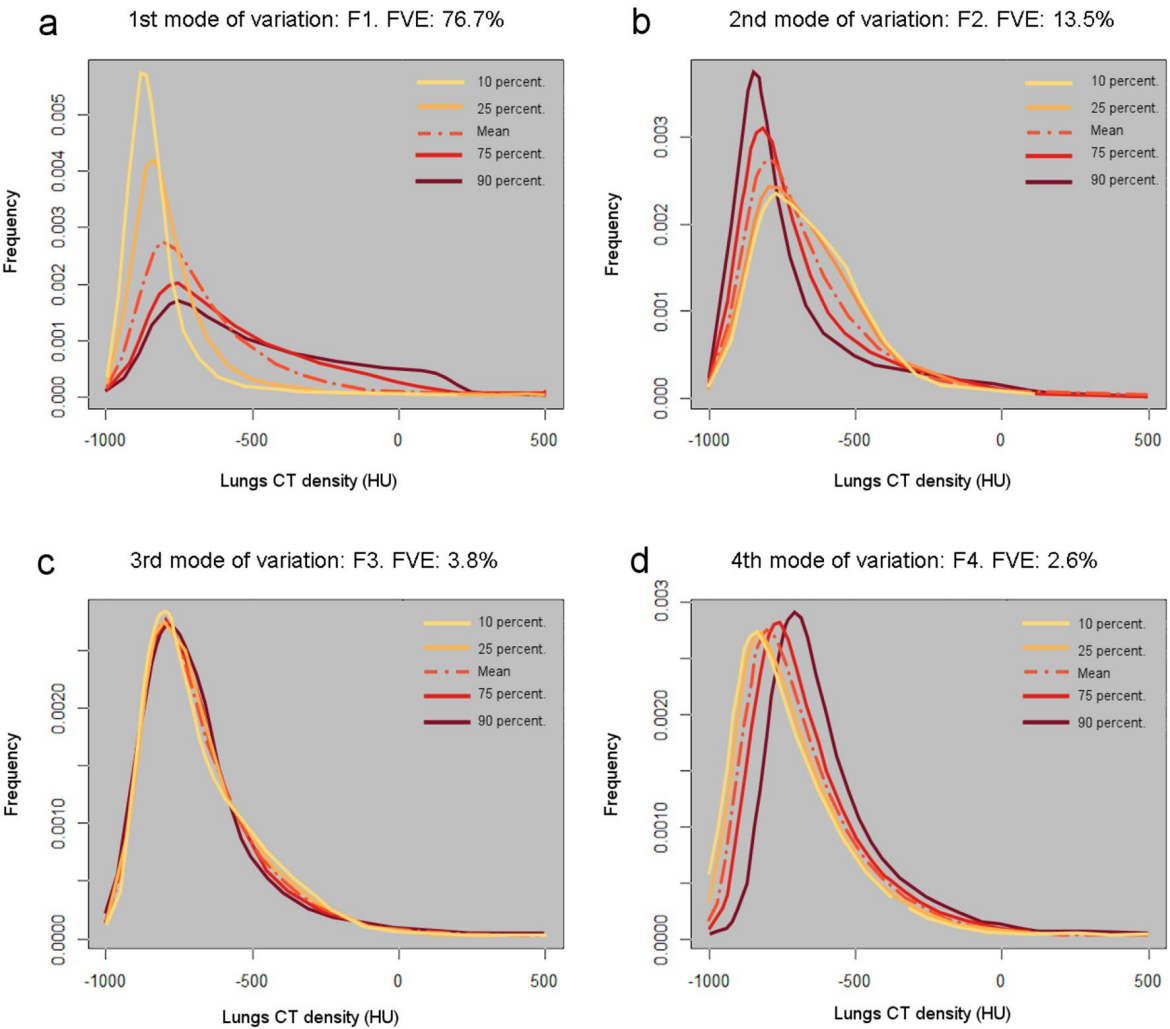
First four modes of variation of the lung CT density curves (smoothed CT histograms). (a) F1 explains 76.7% of the fraction of variance explained (FVE). The curves vary from homogeneous low CT density (10 percentile curve) to heterogeneous higher CT density (90 percentile curve). (b) F2 explains 13.5% of the FVE. The lung CT density vary from homogeneous low density (90 percentile curve) to include mid-low density in the – 700 HU to – 350 HU range (10 percentile curve). (c) F3 explains 3.8% of the FVE. This mode of variation represents a redistribution of CT lung densities from (− 700 HU to – 600 HU) to about (− 600 HU to – 300 HU). (d) F4 explains 2.6% of the FVE. F4 represents a small shift of about 140HU from low homogeneous CT densities (10 percentile curve) toward higher CT densities (90 percentile curve).
